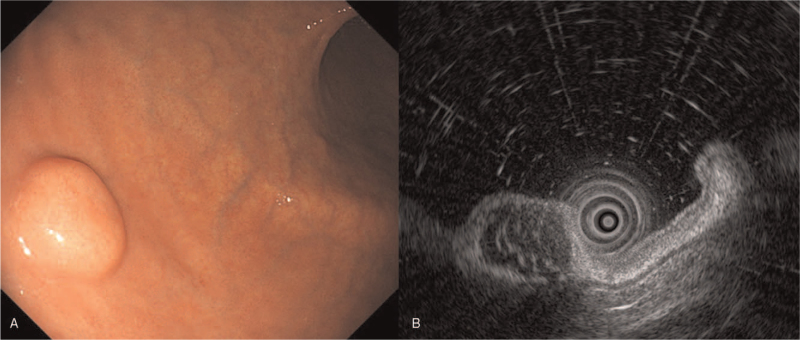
(A) A wide-based and sessile subepithelial tumor covered with normal gastric mucosa is found incidentally on the greater curvature of the stomach body during screening endoscopy. (B) Endoscopic ultrasonography reveals a heterogeneous hypoechoic lesion of 1.4 X 0.5 cm in the submucosal layer.
